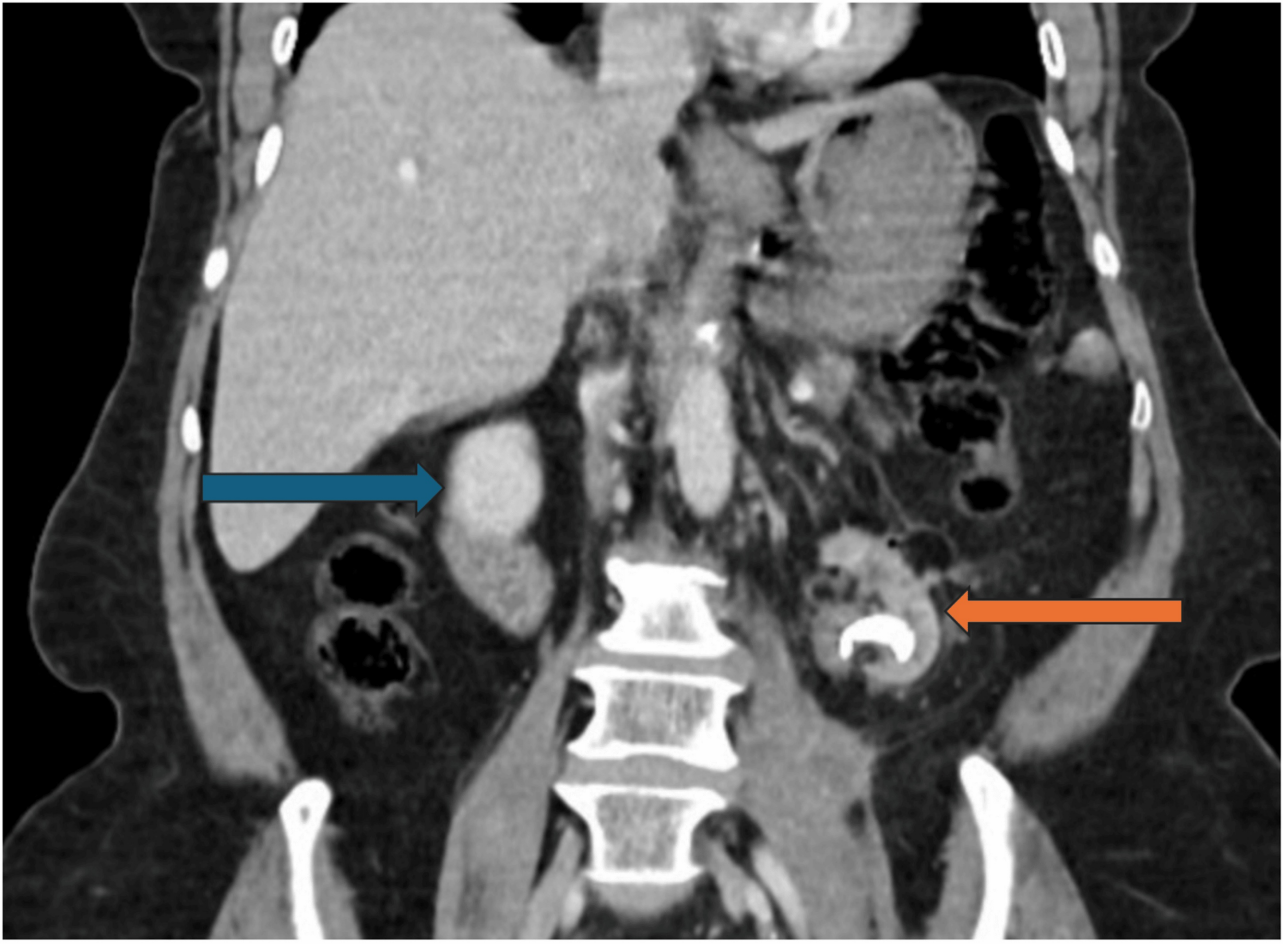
Coronal CT scan showing an atrophic left kidney, indicated by the orange arrow, and a simple cyst in the right kidney, indicated by the blue arrow.
